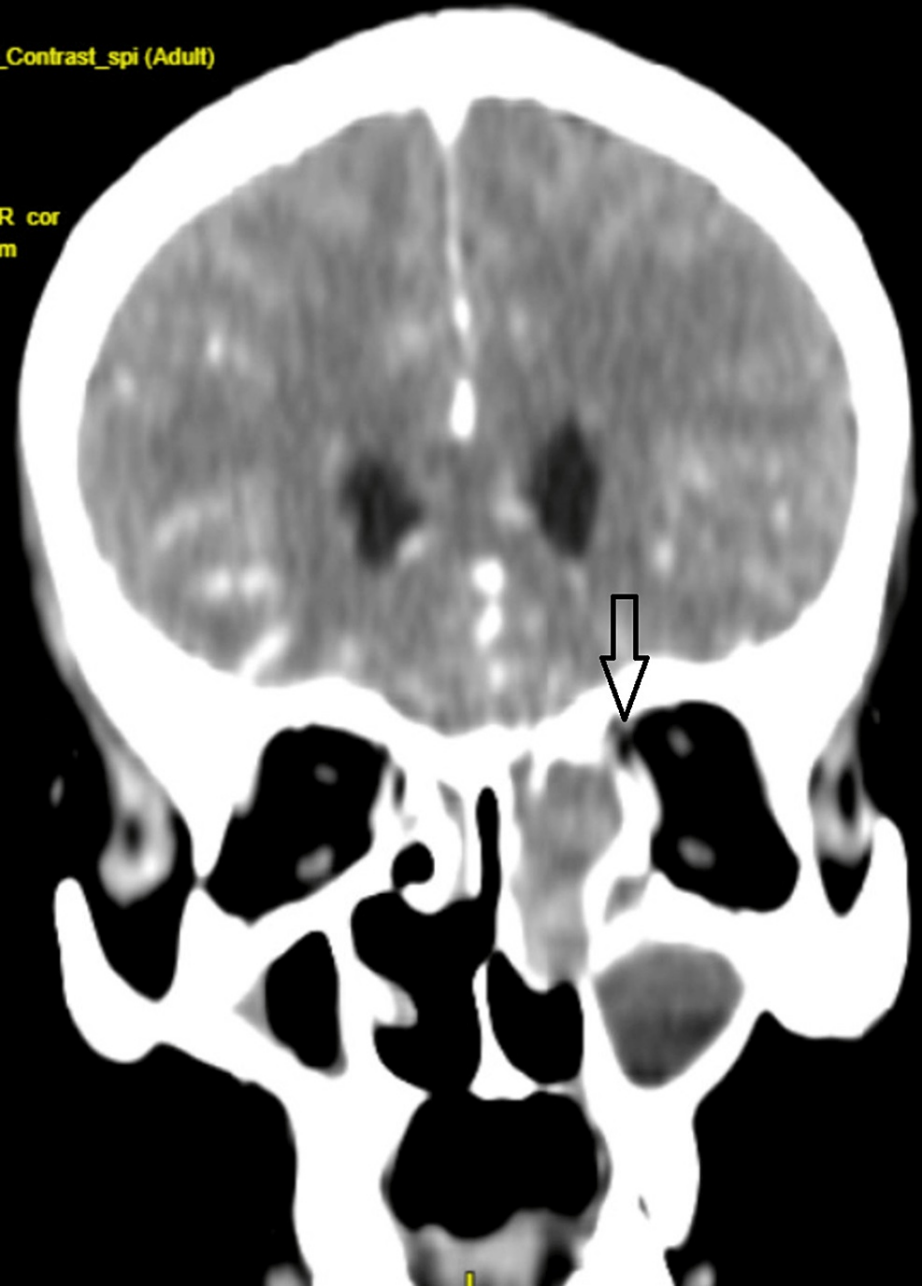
CECT paranasal sinus showing a cortical break in the medial wall of the left orbit (arrow) CECT: contrast-enhanced computed tomography
